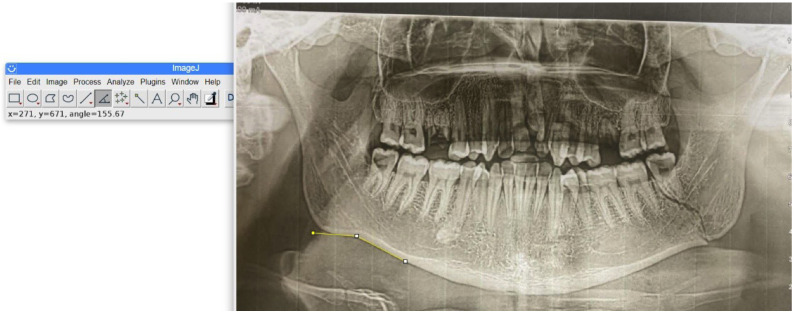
Measuring antegonial angle on digital panoramic radiograph using Image J program.
